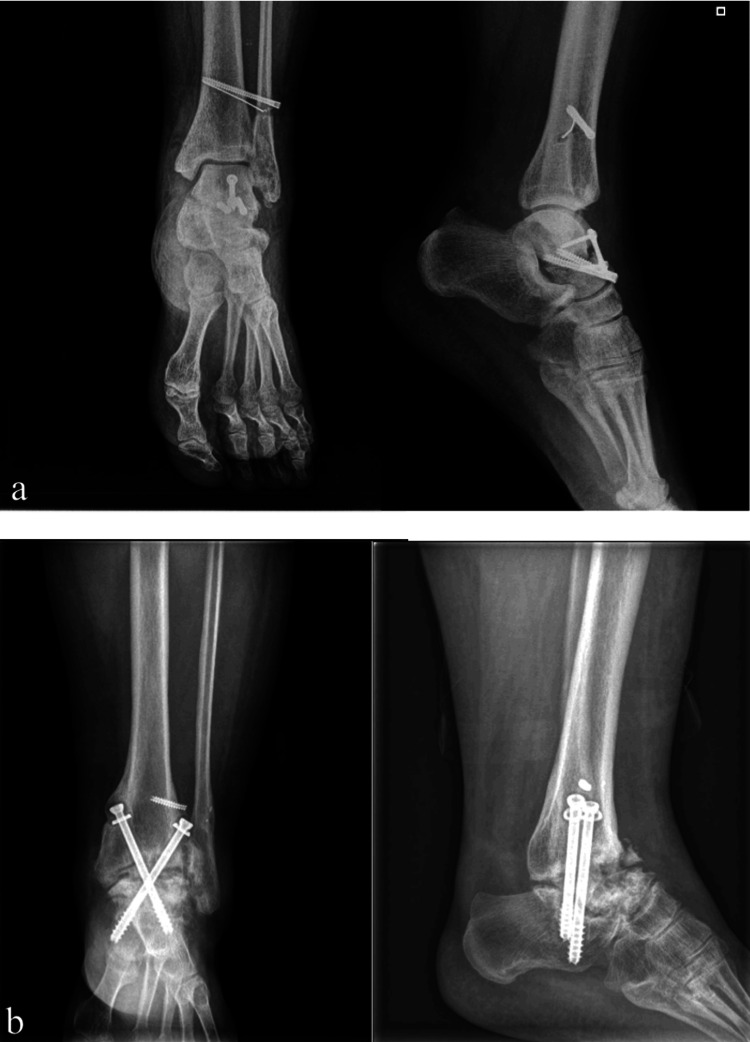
(a) Anteroposterior and lateral X-ray views of the post-traumatic patient showing tibiotalar and subtalar arthritis; (b) 6 months after operation X-ray views showing union by canulated screws
